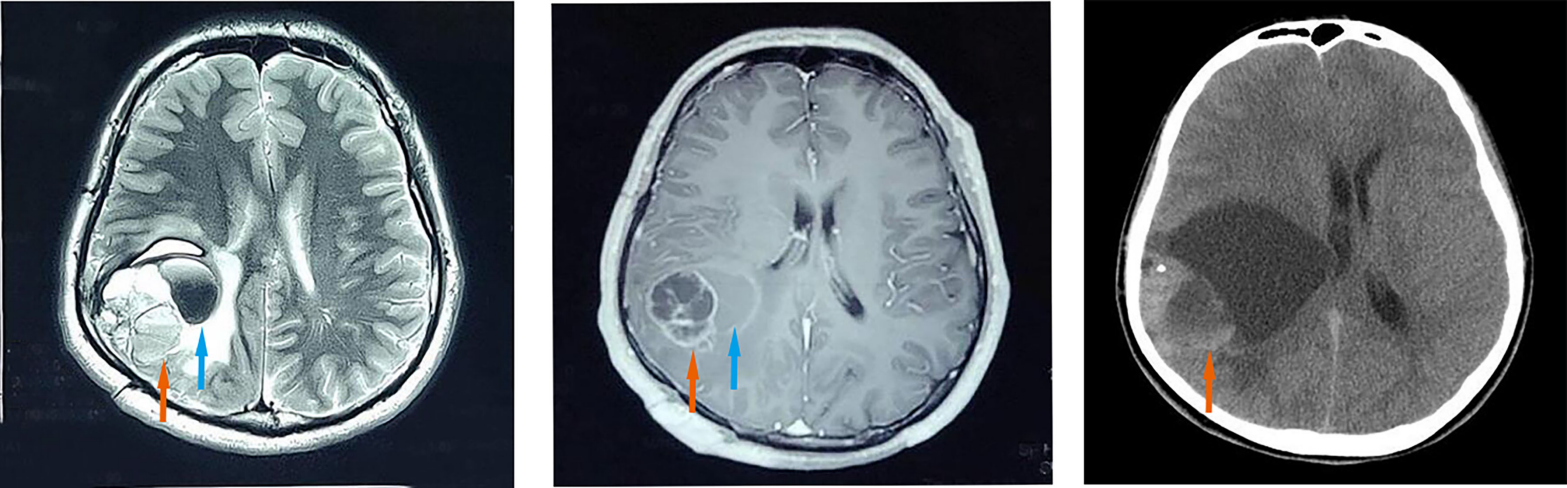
Imaging features. The recurrent lesion appeared as a mixed-signal intensity located in the right temporal-parietal lobe with a polycystic compartment, patchy hypointensity (orange arrow), liquid level inside (blue arrow), and line-like low signal areas at the periphery, surrounded by extensive oedema on axial T2-weighted images (left). Axial contrast-enhanced T1-weighted MR image (middle) demonstrated marked enhancement in the solid portions (orange arrow) of the recurrent mass and no enhancement of the cyst (blue arrow). Axial CT scan of the primary lesion showed a round-like area of mixed density (orange arrow), measuring about 6.3*6.3 cm, in the brain parenchyma of the right parietal lobe with compression and deformation of the right lateral ventricle (right).
